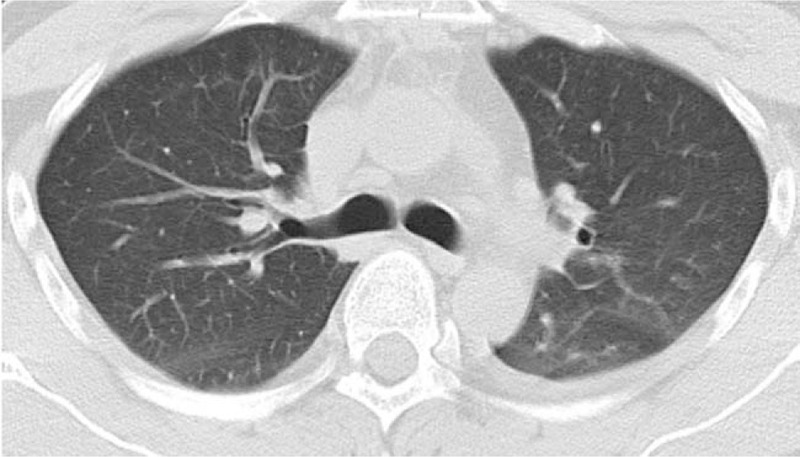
Computed tomographic image on postoperative day 18 (before the patient was discharged). The pulmonary edema is almost resolved.
